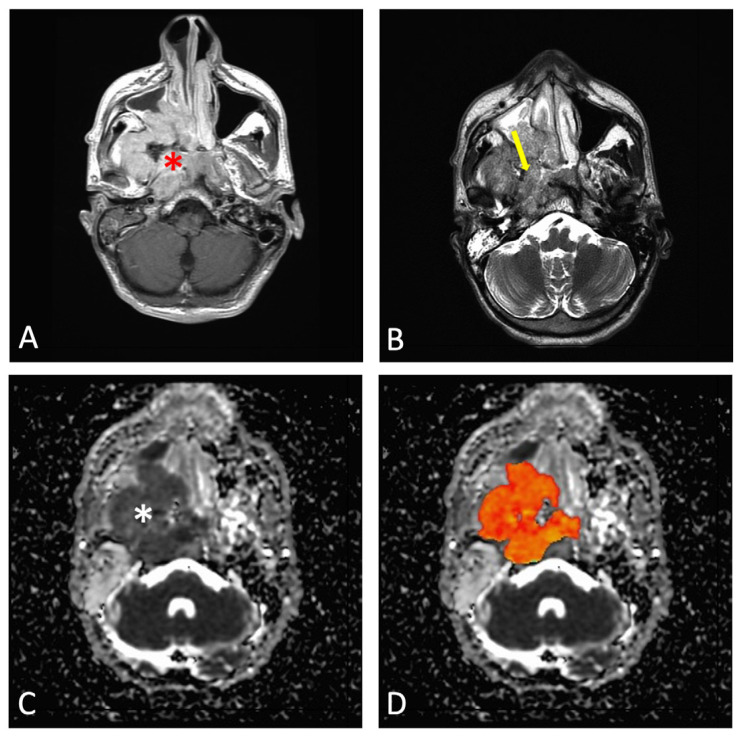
A 43-year-old male with NPC: (A) post-contrast axial T1-weighted imaging showing a large nasopharyngeal mass (red asterisk); (B) axial T2-weighted imaging demonstrating the involvement of the masticatory space (yellow arrow); (C) ADC map showing low signal intensity of the nasopharyngeal mass (white asterisk); (D) ROI was defined by covering as much tumor tissue as possible (shown on the slice with the maximum tumor diameter, orange area).
